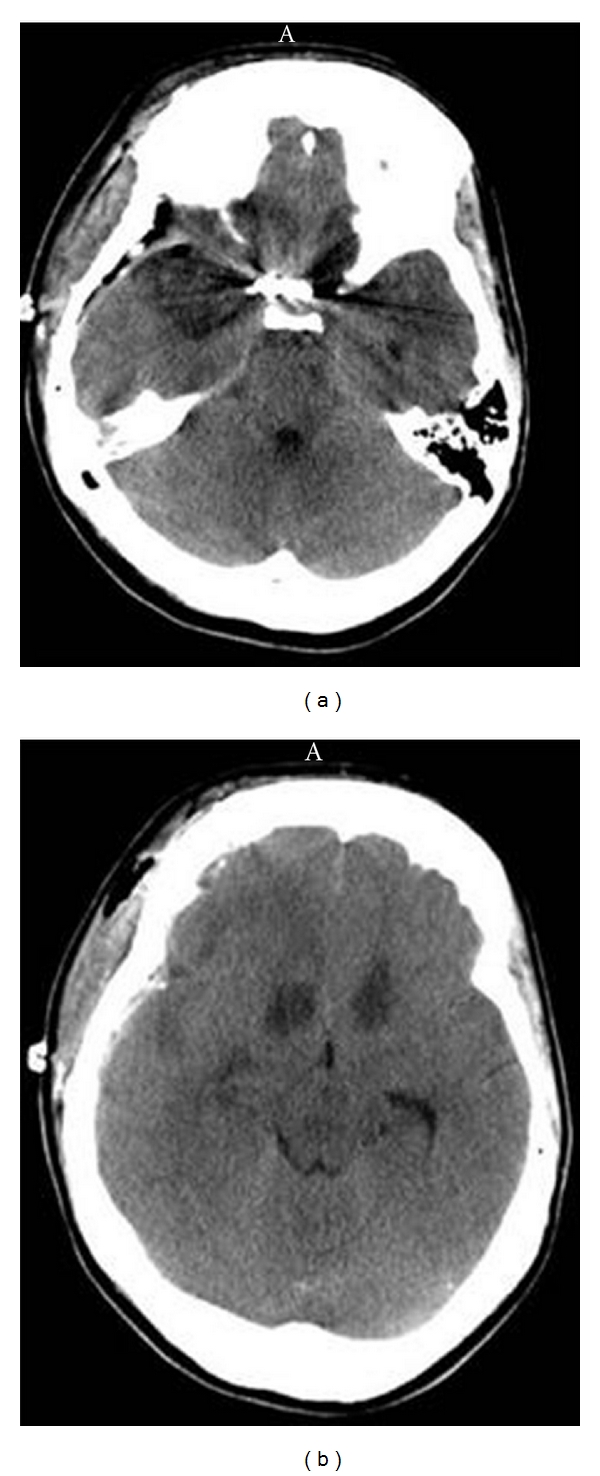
Participant no. 3 with a right frontotemporal lesion. The CT image in (a) depicts a large lesion within the right anterior inferior medial temporal lobe with incursion of the lesion to include both straight gyri of the orbitofrontal lobes. The CT image in (b) depicts bilateral lesions in the cortex underlying the anterior-inferior cingulate gyri. Although the lesions are bilateral, (and this poses some degree of difficulty in making firm conclusions), the lesions are significantly larger and of greater extent in the right hemisphere.
